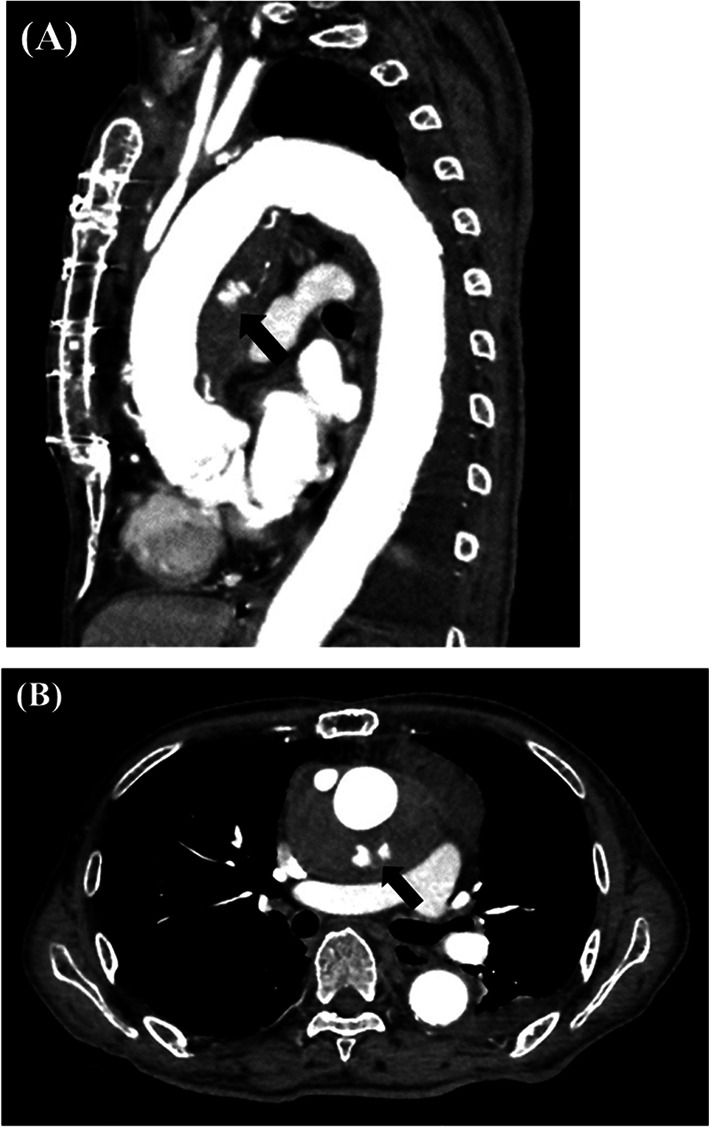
Contrast-enhanced CT findings. An intra-mediastinal hematoma around the vascular graft of the ascending aorta and extravasation on the dorsal side of the center of the vascular graft (black arrows). A Sagittal view; B Axial view
